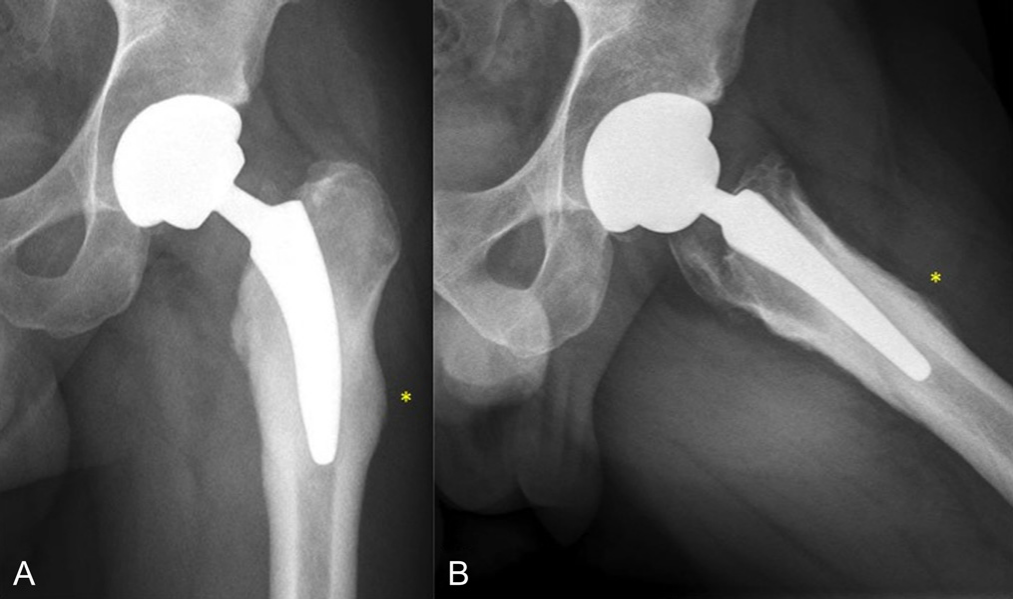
A and B. Left hip's anteroposterior and lateral radiographs at 2 and half years of follow-up, evidencing a hypertrophic callus (*) with no signs of stem loosening, being the patient completely asymptomatic.
